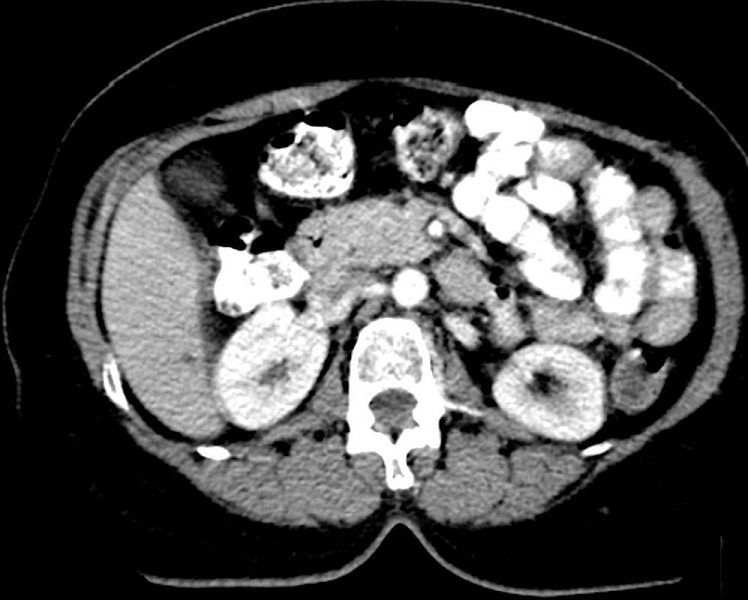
Axial computed tomographic scan performed two years after resection showed no evidence of tumour recurrence
